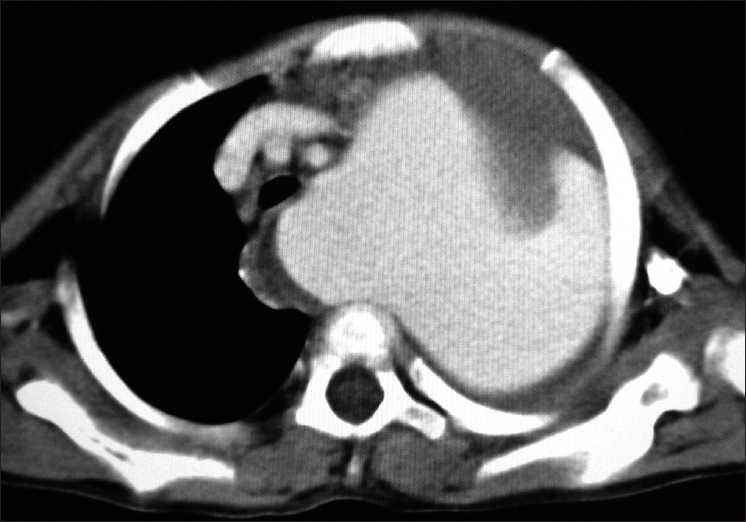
HRCT chest (lung window) showing multiple nodules, halo sign (thick, short arrow) and pneumatoceles (thin, long arrow)
